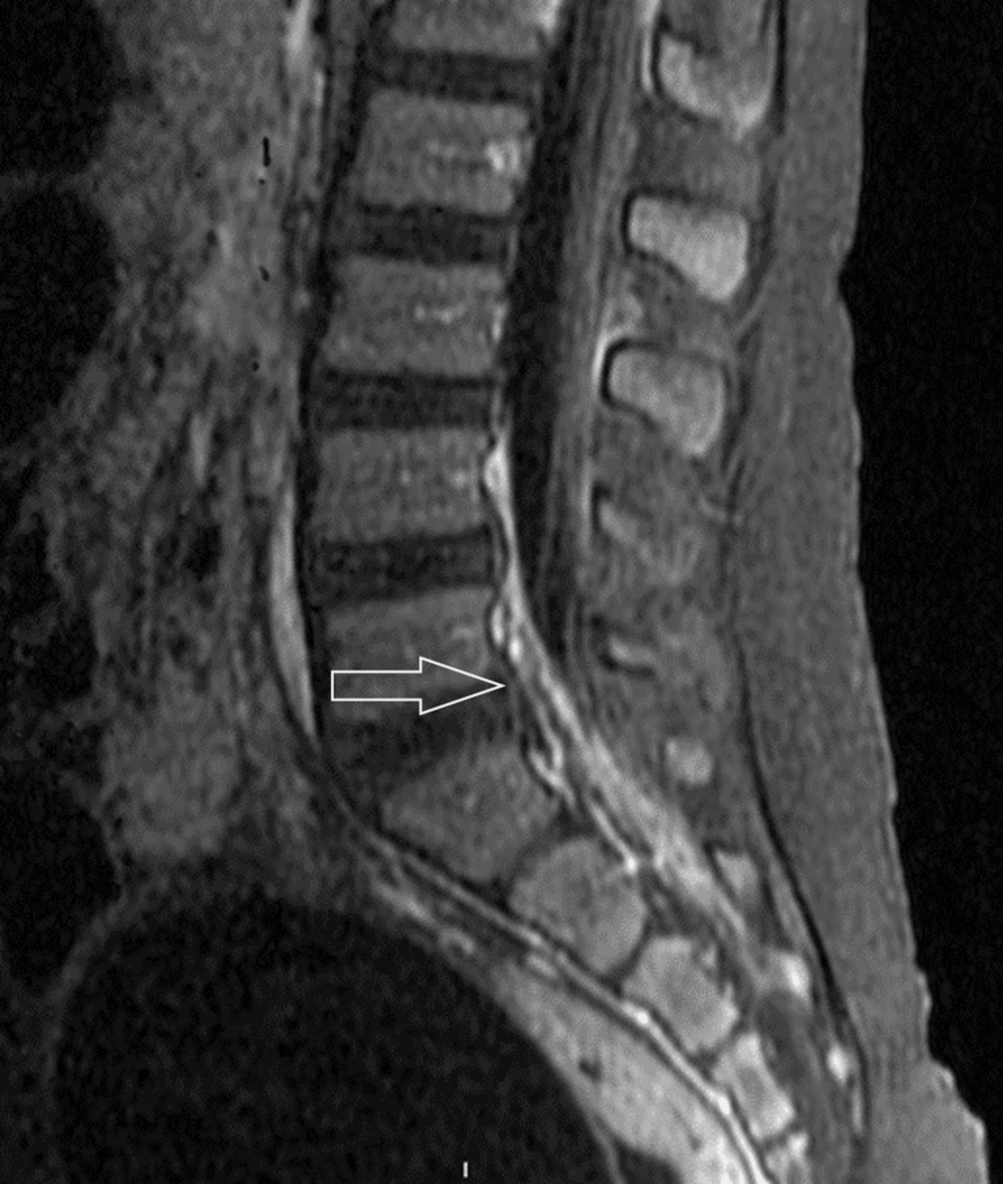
MRI showing prominent enhancement of the ventral epidural space of L5-S1 representing the developing phlegmon (arrow) MRI: magnetic resonance imaging
